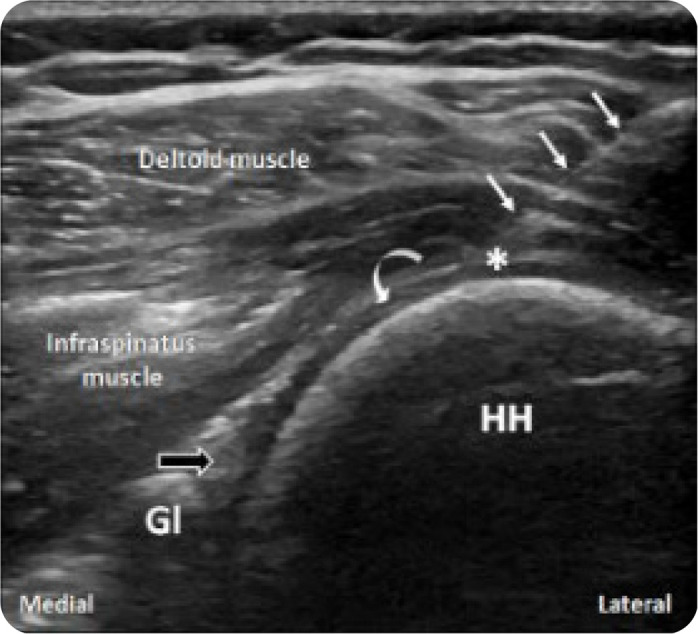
Sonographic image along the long axis of the infraspinatus tendon showing needle advancement (thin arrows) to the target point (*) immediately superficial to the articular cartilage (curved arrow) of the humeral head (HH). The glenoid (Gl) and the posterior labrum (black arrow) can be seen at the level of the posterior joint recess.
